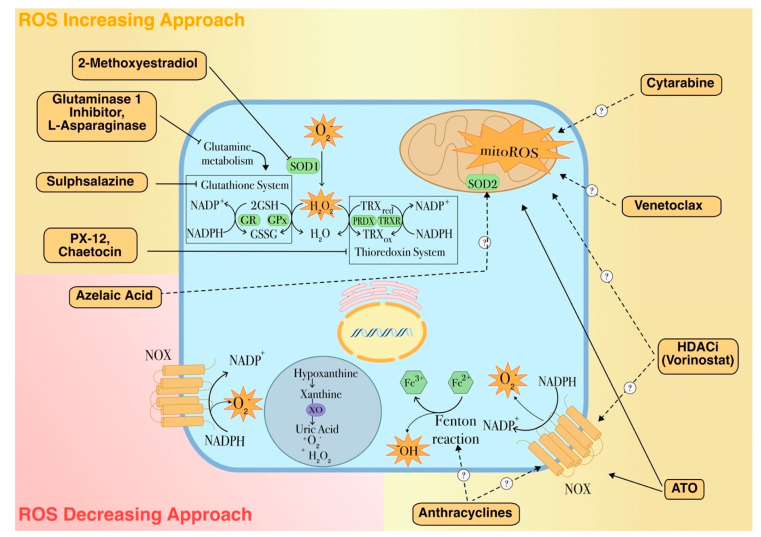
ROS-dependent therapeutic agents for leukemia treatment. The mechanism of action and the molecular targets of pro- or antioxidant drugs used in leukemia are shown. Along with conventional treatments, this figure depicts some promising examples of these approaches. 2-Methoxyestradiol (2ME2), a naturally occurring estrogen metabolite with antiproliferative and antiangiogenic activities is able to induce apoptosis through a ROS-dependent mechanism. Also, this drug is able to target LSCs by inhibiting the transcriptional activity of HIF-1α, that is found over-expressed in LSCs under hypoxic conditions, thus down-regulating pro-leukemic HIF-1α target genes, including the vascular endothelial growth factor (VEGF) [130,131]. L-asparaginase treatment has recently been reported to induce autophagy by promoting apoptosis and cell growth inhibition in AML cells and has synergistic effects with conventional AML chemotherapies [132]. The thiodioxopiperazine natural product chaetocin (SUV39H1 inhibitor) is a competitive substrate and inhibitor of thioredoxin reductase and in this way induces cellular oxidative stress. In addition, as inhibitor of SUV39H1, a co-factor of the transcription factor RUNX1 which has an important role in the regulation of proliferation and self-renewal of hematopoietic stem cells, chaetocin also promotes differentiation of AML cells and has synergistic effects with HDAC inhibitors [133,134]. Dotted arrows with question marks indicate plausible mechanisms of action. Full and dashed arrows indicate well-established or hypothetical molecular mechanisms, respectively.
